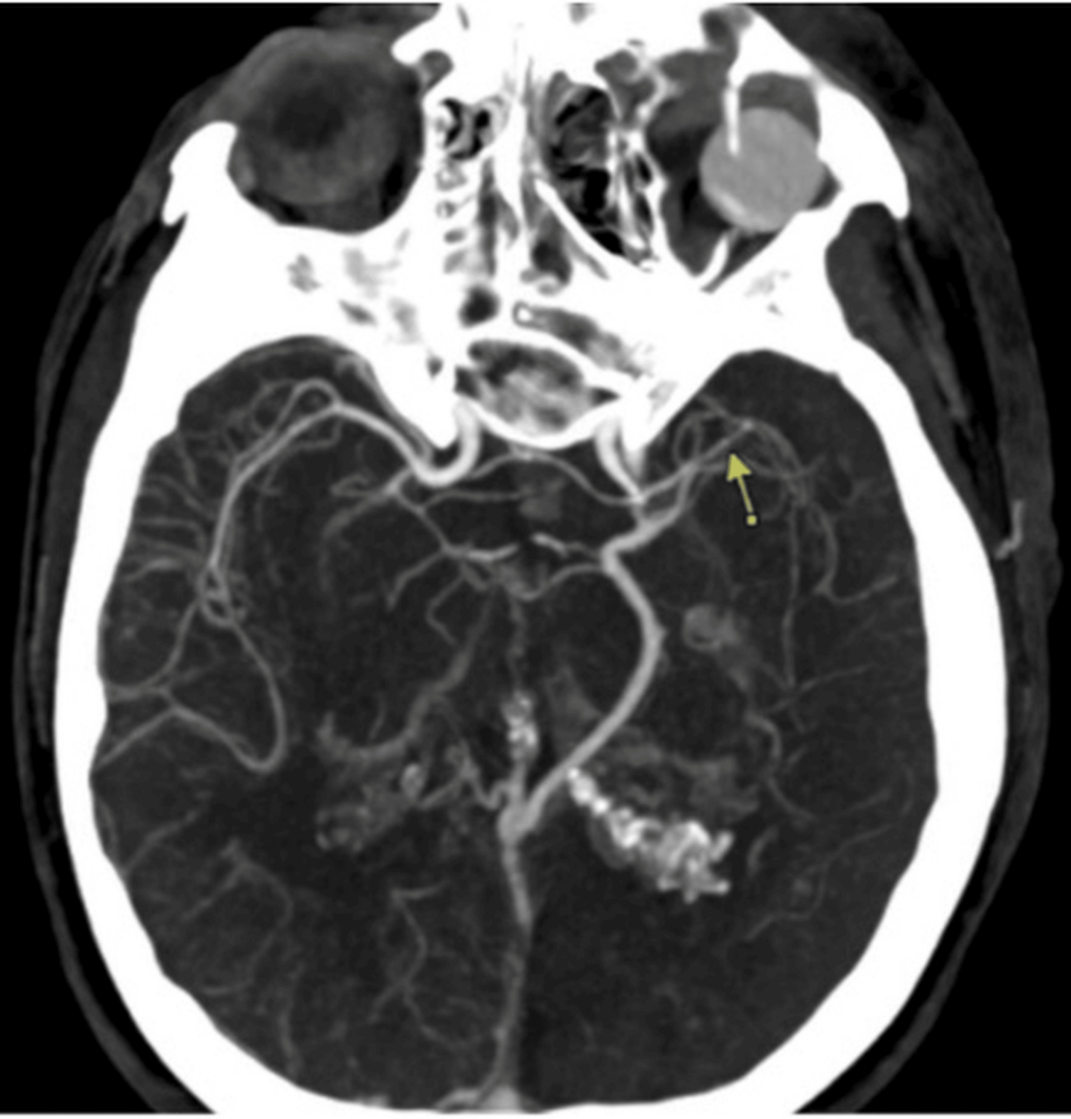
CT angiogram of the head showing new moderate-to-severe diffuse narrowing of the left middle cerebral artery (arrow)
